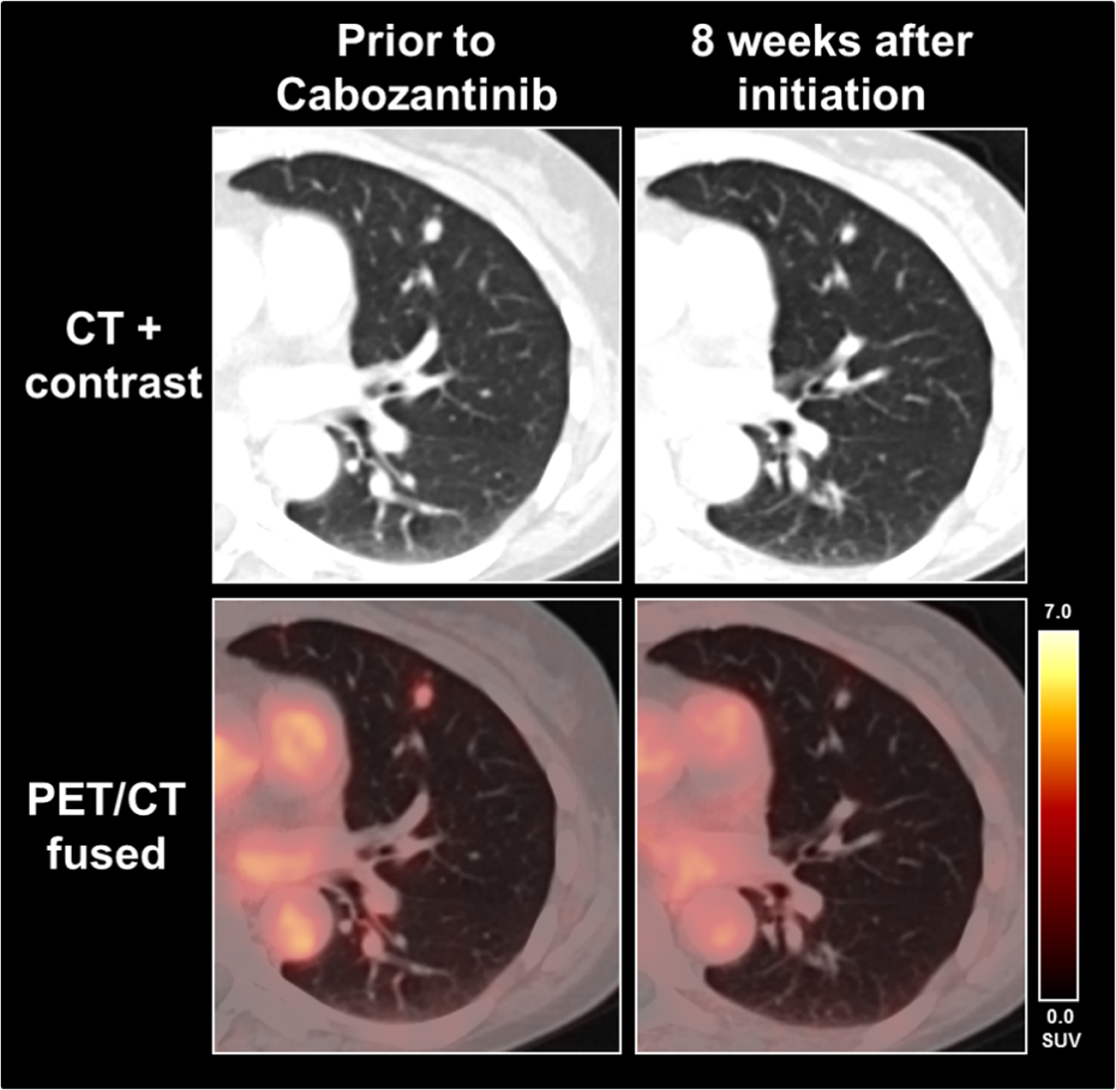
A 53-year-old female patient showed a slightly decreasing pulmonary metastasis, which, however, completely lost PSMA expression during therapy with cabozantinib
